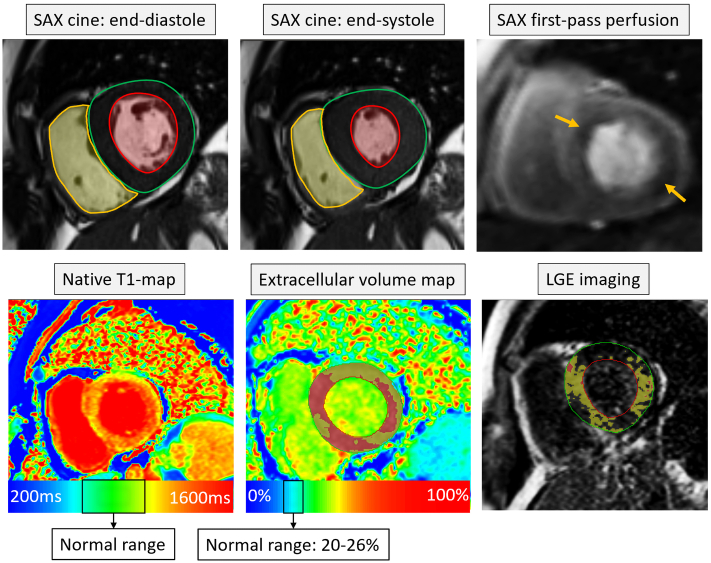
Multiparametric cardiac magnetic resonance imaging in patients with HFpEF. In this particular case, there is evidence of concentric left ventricular hypertrophy with an LV ejection fraction of 62%. Also, there is evidence of perfusion defect during first-pass perfusion (orange arrows). T1-mapping shows a global pattern of rise in values. ECV mapping demonstrates large areas of the myocardium with an ECV of 30% to 50% (yellow area) and also >50% (red areas of the myocardium). A similar pattern of fibrosis was seen on LGE imaging. (For interpretation of the references to colour in this figure legend, the reader is referred to the web version of this article.)
